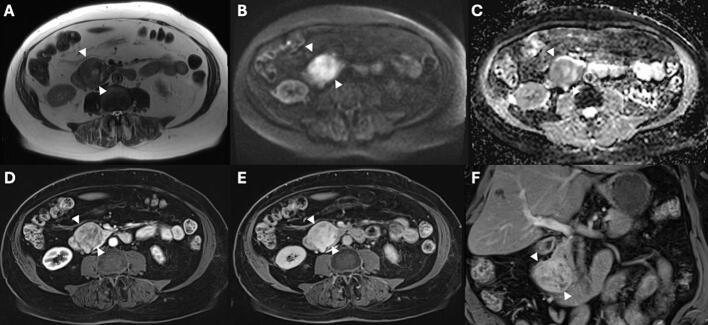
Abdominal MRI in axial (A-E) and coronal (F) direction. A mass in the pancreatic head (arrowheads) with a maximum diameter of 49 mm is observed, with a heterogeneous hyperintense signal intensity on T2 weighted imaging (A), and clear diffusion restriction (B and C), as well as a heterogeneous enhancement pattern in the late arterial (D) and portal venous phase (E and F).
